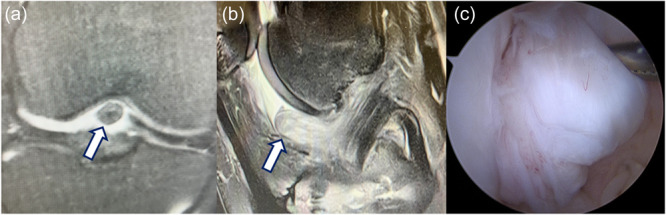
Cyclops syndrome identified 9 months after anterior cruciate ligament reconstruction. (a) Frontal plane magnetic resonance imaging (MRI). (b) Sagittal plane MRI. (c) Arthroscopic view of the fibrous nodule within the intercondylar notch. Arrows indicate the cyclops lesion.
